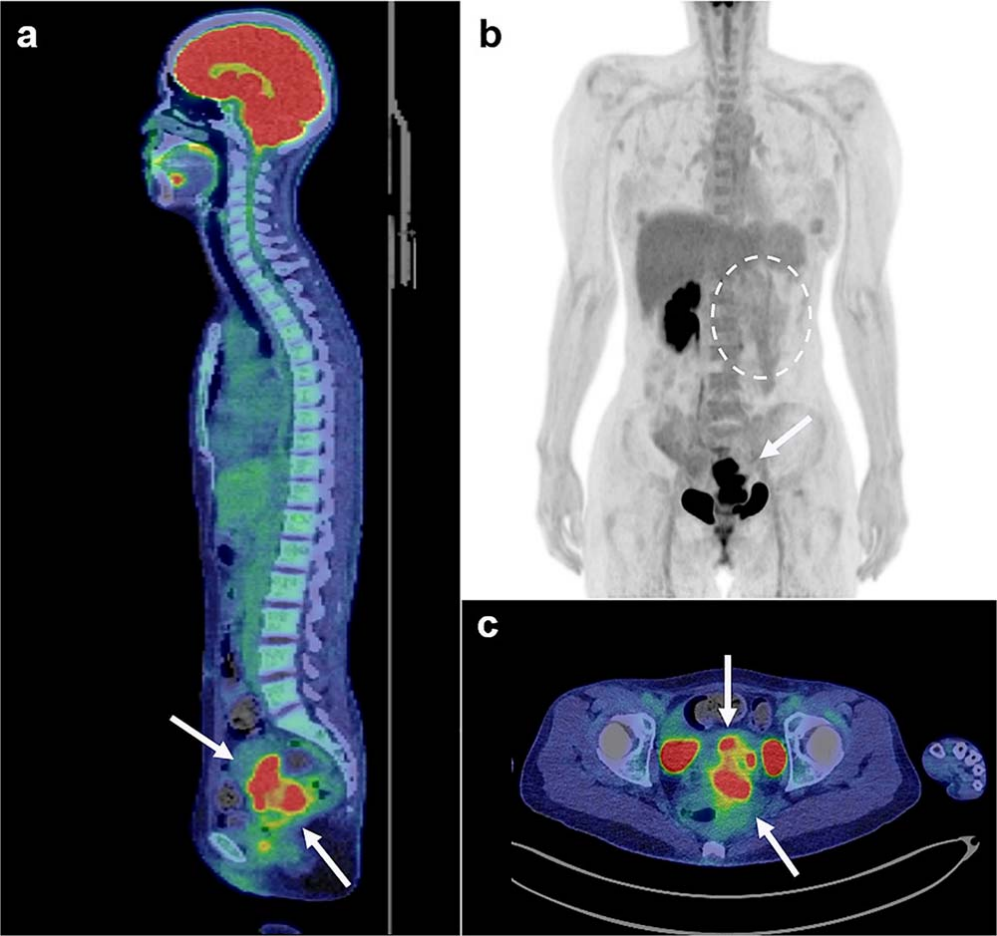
FDG positron emission tomography and computed tomography scans (FDG PET/CT). FDG PET/CT revealed that the mass that had FDG uptake solely in the pelvis (a, b, c: arrows). The left kidney did not uptake FDG because it was non-functioning (b: circle with dotted line).
